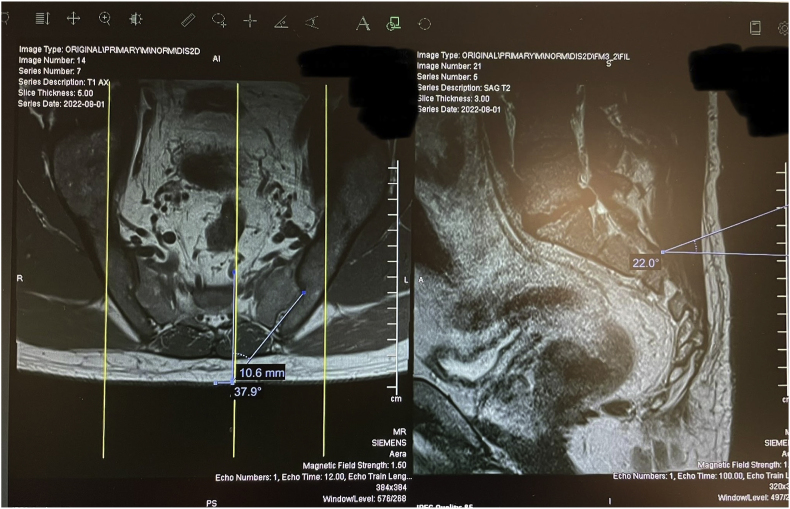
Axial and Mid-sagittal MRI images of SIJ with bilateral varus angulations. This figure depicts an example where the contralateral oblique angle is 37.9° (left image) and Cranial-Caudal tilt angle is 22° (right image).
